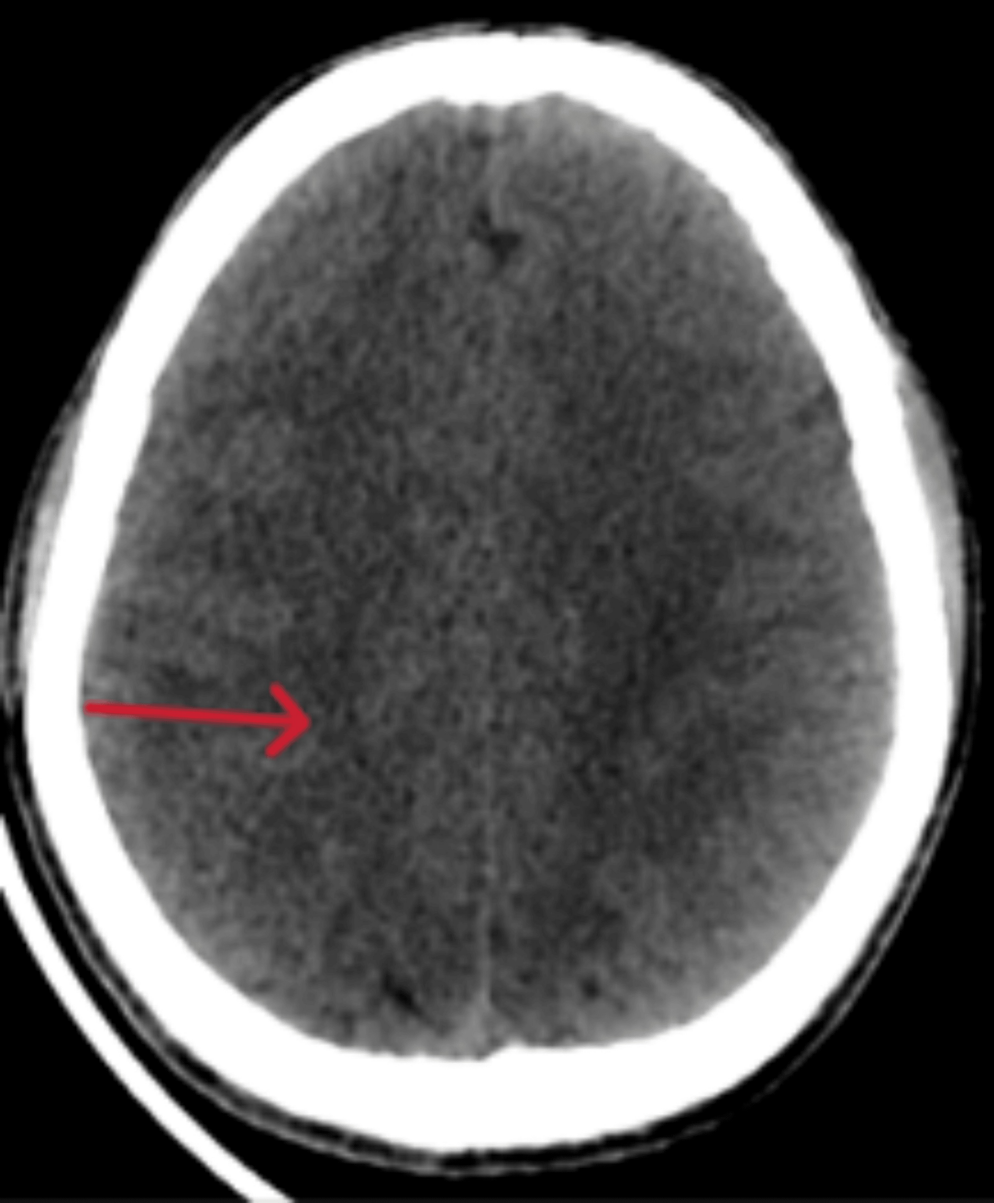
Trans axial CT scans passing through the high parietal cortex showing diffuse sulcal effacement indicating mild cerebral edema. CT: Computed tomography
